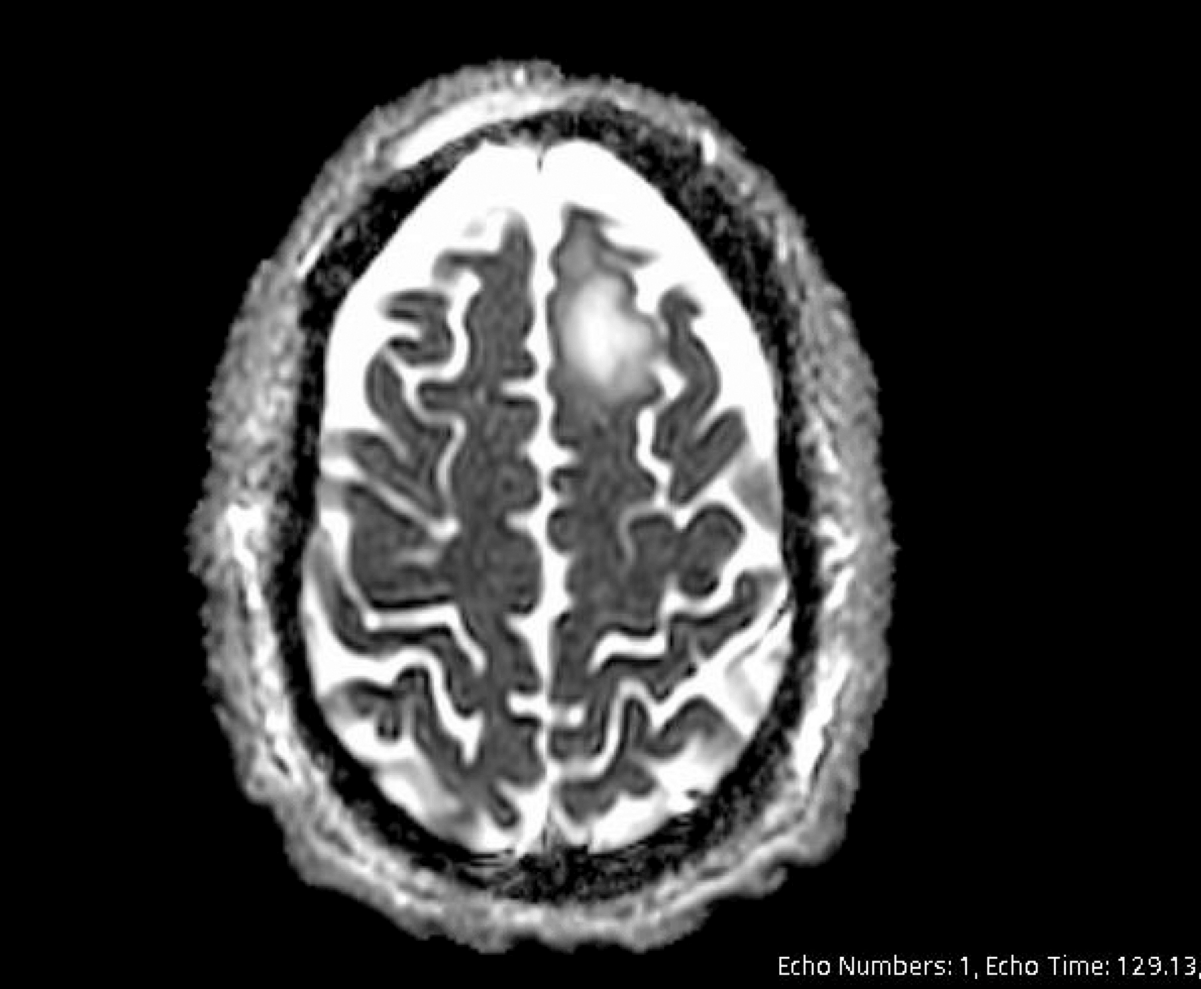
Patient's MRI of the head without contrast demonstrating a left frontal lobe lesion as described above.
